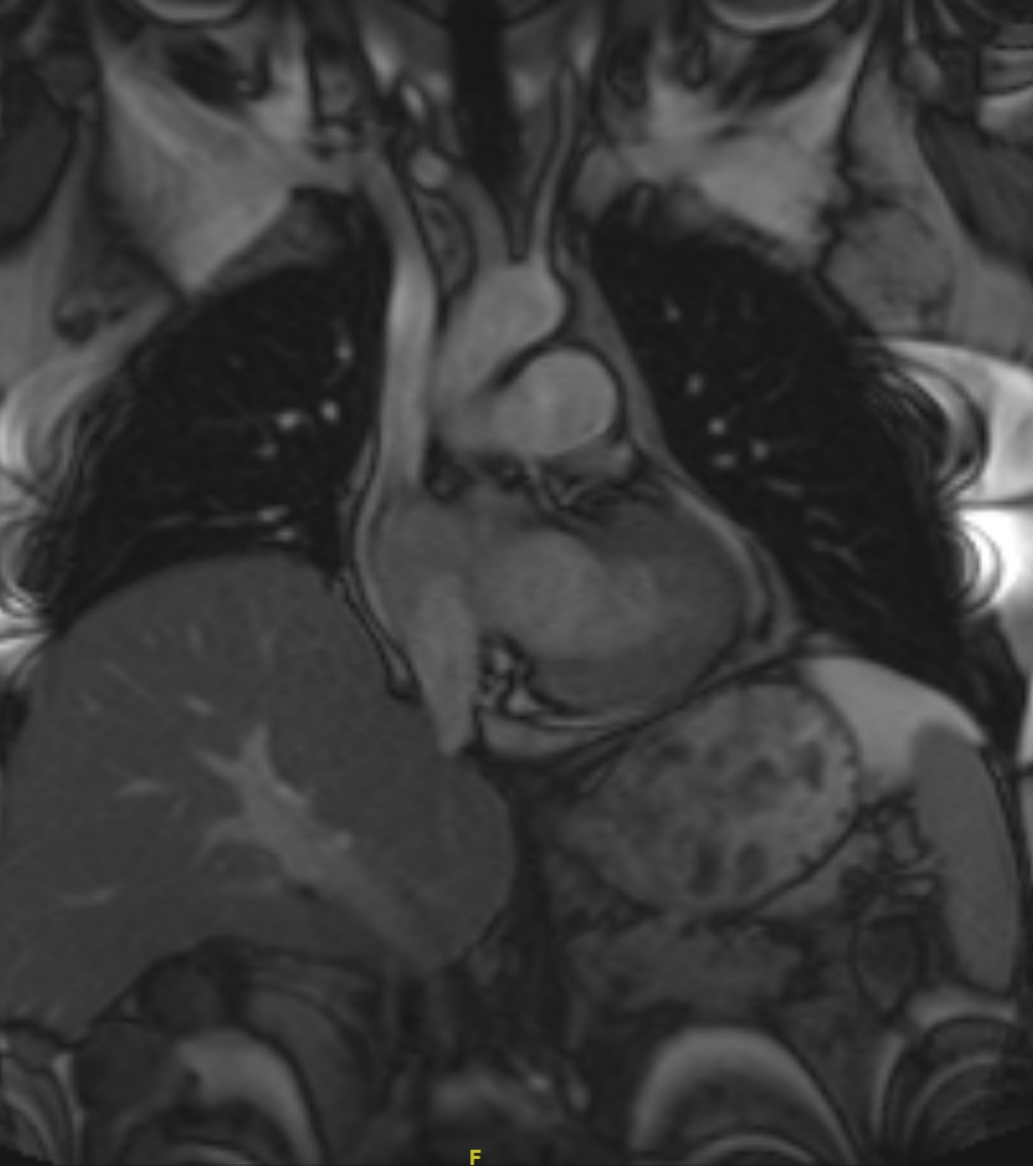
Cardiac Involvement with Merkel Cell Carcinoma Cardiac MRI showing involvement of the myocardium with Merkel cell carcinoma.
